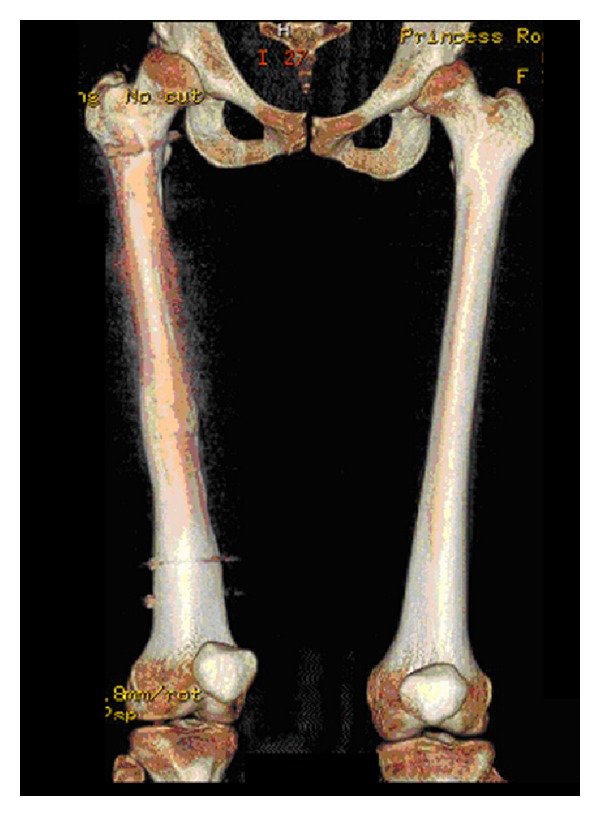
CT reconstruction showing rotational deformity of the right femur.
